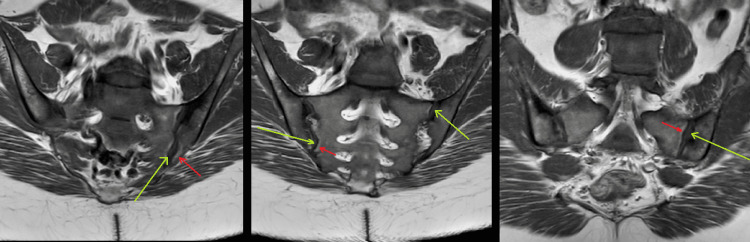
MRI of the sacrum revealed mild widening of the sacroiliac joints (long arrows) with periarticular sclerosis (short arrows).
